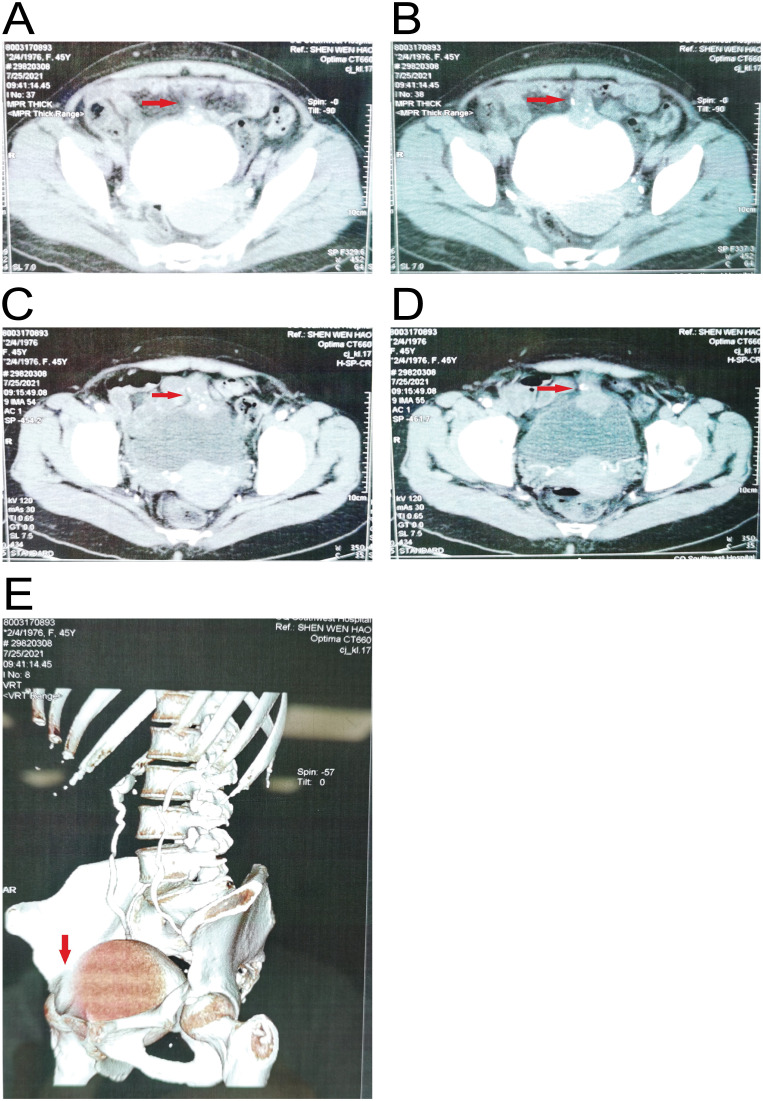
Images of the patient before operation. (A, B) The anterior wall of the bladder exhibits irregular soft tissue density, and the tumor extends into and out of the cavity. Calcifications are seen in the tumor area, and the maximum cross-sectional area of the tumor is about 4.9*3.1cm; (C, D) Enhanced CT shows mild inhomogeneous enhancement. The excretory stage shows a filling defect, and there is no obvious enlarged lymph node shadow around. (E) CT three-dimensional imaging of the ureter shows a space-occupying lesion in the anterior wall of the bladder.
